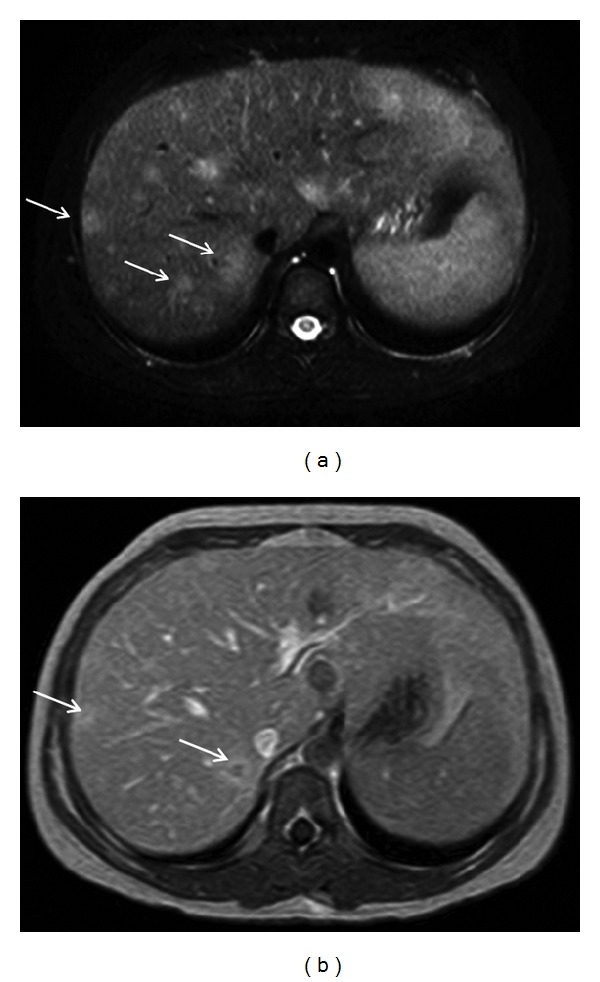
Abdominal MRI, axial images through the liver: (a) STIR sequence reveals multiple high intensity focal lesions (arrows) with indistinct borders due to perilesional edema in the liver; the lesions demonstrate peripheral enhancing rim in the T1W images after administration of IV contrast medium (arrows in (b)).
